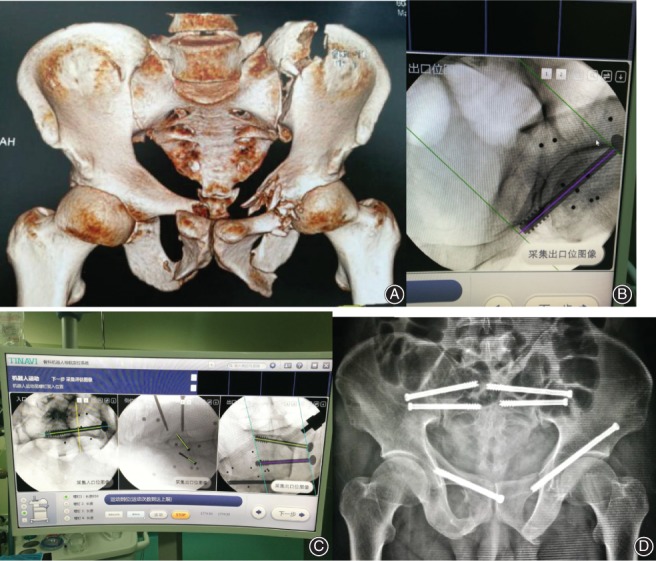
Male, 47 years old, car accident injury, bilateral iliac and pubic rami fractures, treated with robot‐assisted percutaneous cannulated sacroiliac screw fixation and pubic ramus screw fixation. (A) Preoperative CT 3D reconstruction. (B) Path planning of pubic ramus screw placement. (C) Path planning of sacroiliac screw placement. (D) Postoperative pelvic anteroposterior image.
